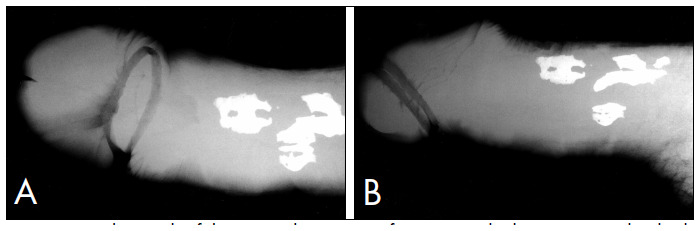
Radiograph of the penis showing ossification inside the septum and in both corpora cavernosa. A: frontal view; B: sagittal view.
